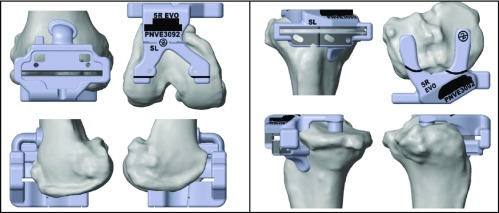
Femoral and tibial cutting block mounted on the distal femur and on the proximal tibia respectively. These are company manufactured patient specific femoral cutting blocks based on CT scan created virtual bone models. Multiple contact points on the femoral cutting block and the tibial cutting block, along with markings allow accurate positioning and rotational alignment improving accuracy of the cuts and femoral and tibial rotation respectively. There are slots for external jigs to double check slope and tibial base plate rotation.
